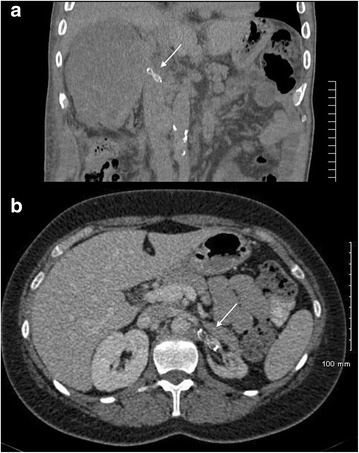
a Coronal image of an unenhanced CT showing the device (*white arrow*) in situ in the right renal artery. b Axial contrast-enhanced CT image from another patient showing the device (*white arrow*) in situ in the left renal artery
